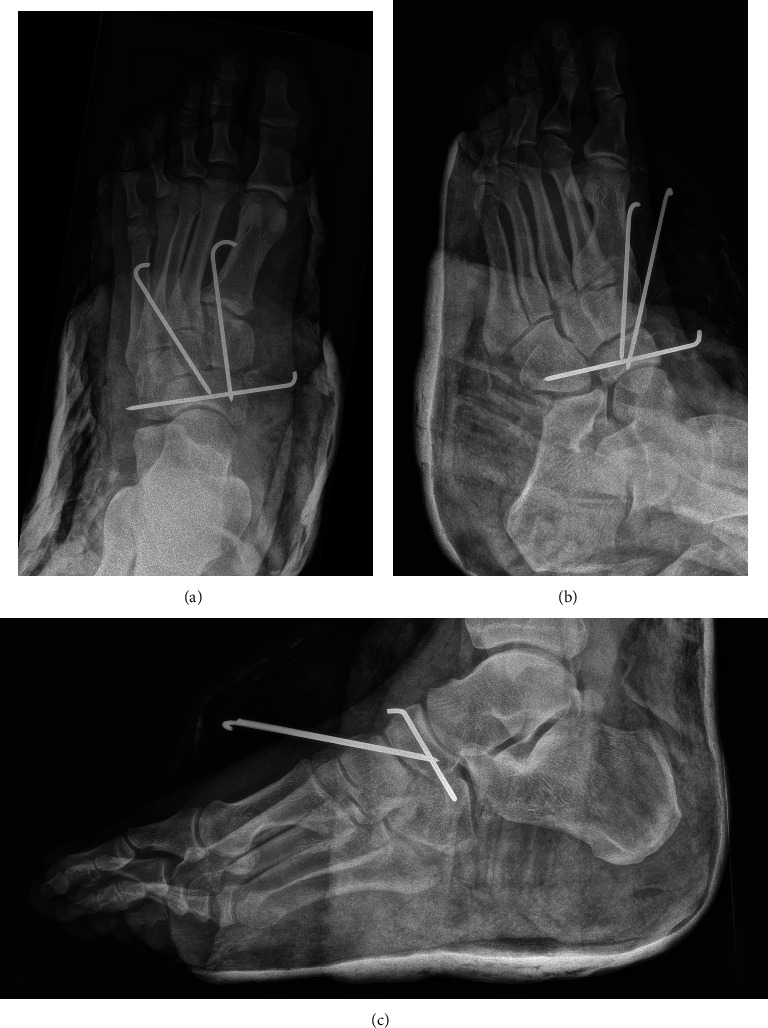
(a) Anteroposterior, (b) oblique, and (c) lateral X-rays of the left foot after closed reduction and percutaneous pinning.
